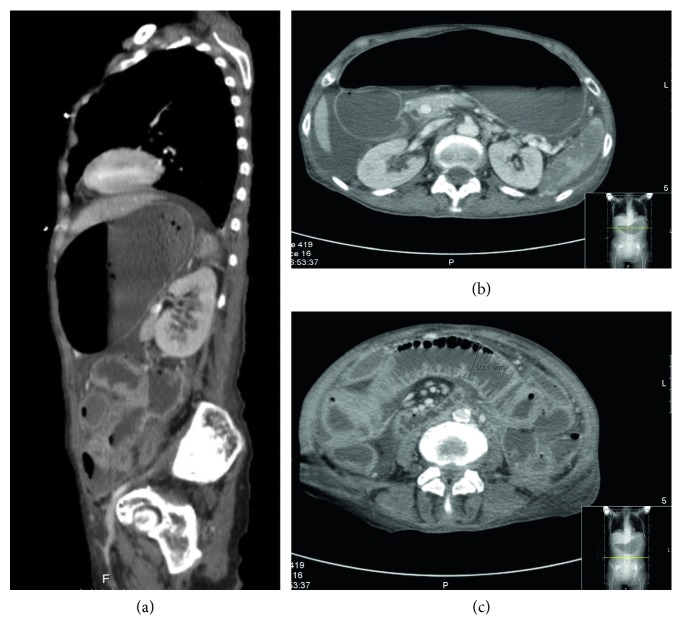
Thoracic, abdominal, and pelvic CT from the day of admission in (a) sagittal and (b, c) transverse planes.
